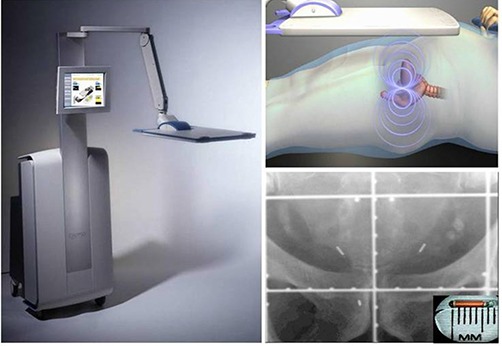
The Calypso console (left) with touch screen monitor and transmitter / receiver array, which generates a resonant signal in 8mmlong×2mm diameter transponders (top‐right) implanted in the right and left base and the apex of the prostate (bottom‐right). The implantation is done under the guidance of ultrasound in a manner similar to a needle biopsy.
